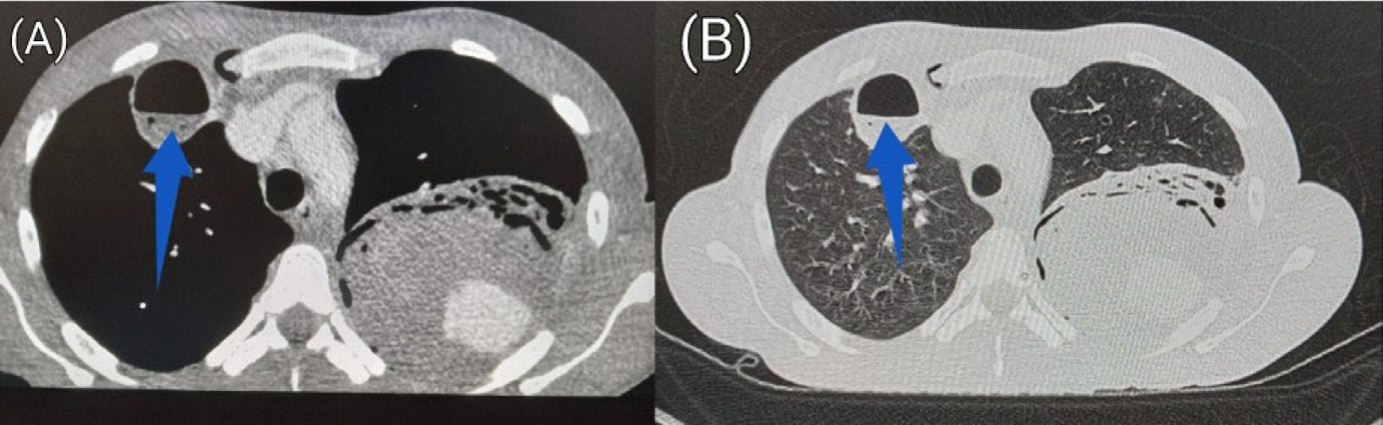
Post‐contrast axial CT sections of the chest in soft tissue (A) and lung (B) window demonstrating a small cavity (blue arrow) with air‐fluid level in the right upper lobe.
